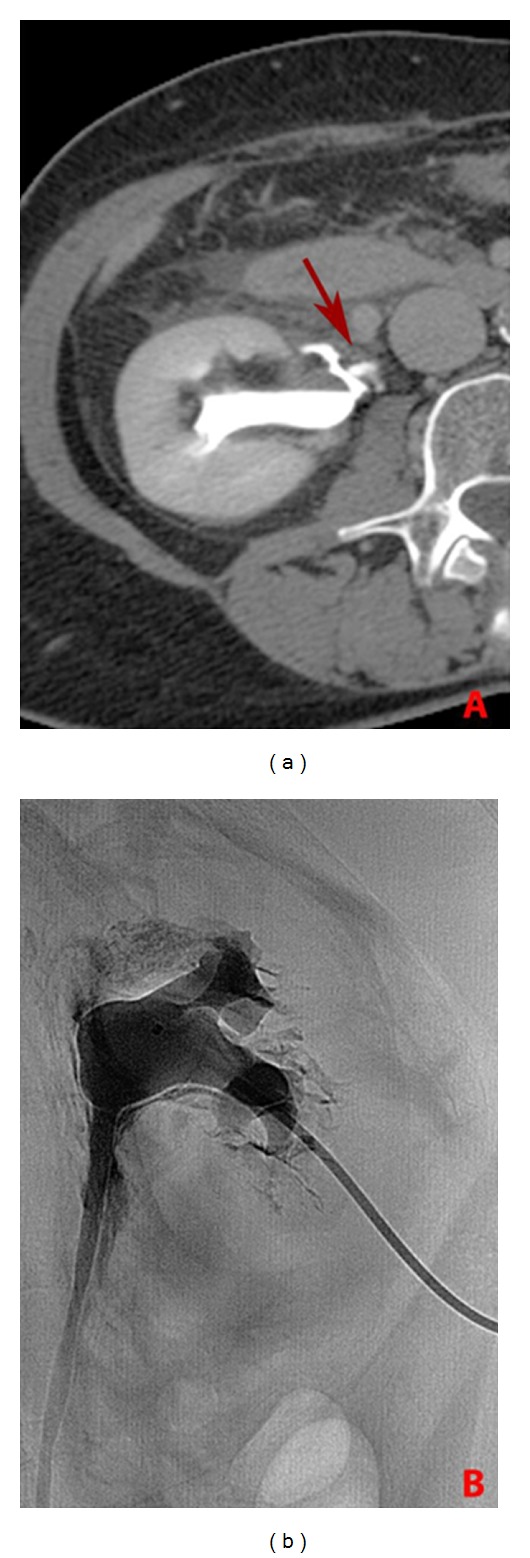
Axial contrast enhanced CT image (a) and fluoroscopic view (b) showing the site of rupture of the excretory system (arrow).
